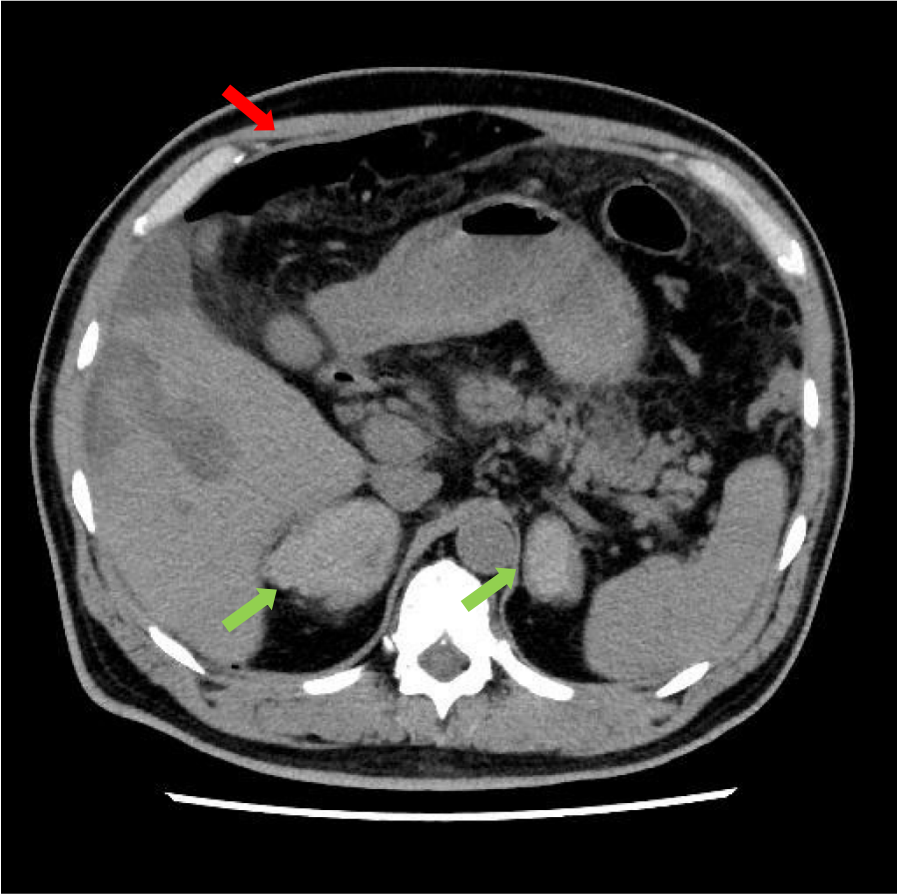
Plain computed tomography findings on day 10. Plain computed tomography scan shows some hypodense lesions in the liver, bilateral adrenal enlargement with high-attenuation (green arrows), and free air in the right side of the upper abdomen (red arrow)
